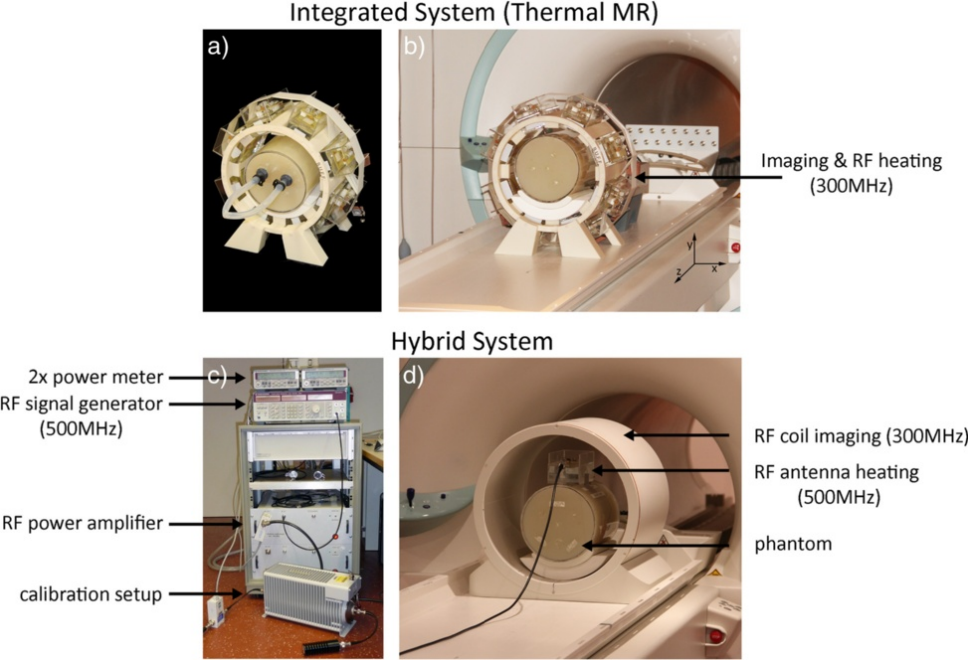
a-b Experimental setup of an 8-channel thermal MR applicator comprising an array of bow tie dipole antennas that support MR imaging, MRTh and targeted RF heating. c-d Experimental setup of a hybrid system using an external RF signal generator and RF amplifier at 500 MHz for RF heating, while MRI and MRTh is being performed at 7.0 T with a RF coil operated at 300 MHz. The power amplifier was calibrated to provide an output of 70 W at the end of the feeding cable. A power meter was used to monitor forward and reflected power during the RF heating experiments
